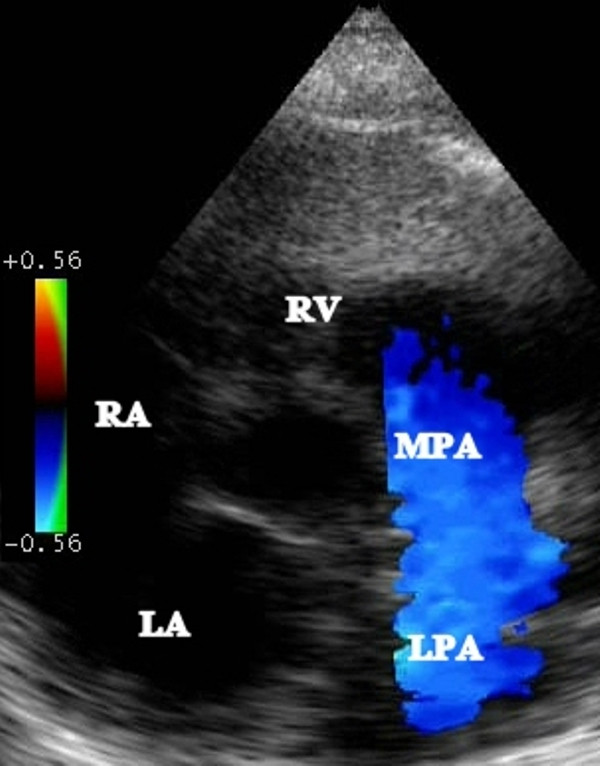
Parasternal short-axis color Doppler view of the right ventricular outflow tract showing the absence of the right pulmonary artery (LA: left atrium, RA: right atrium, RV: right ventricle, MPA: main pulmonary artery, LPA: left pulmonary artery).
